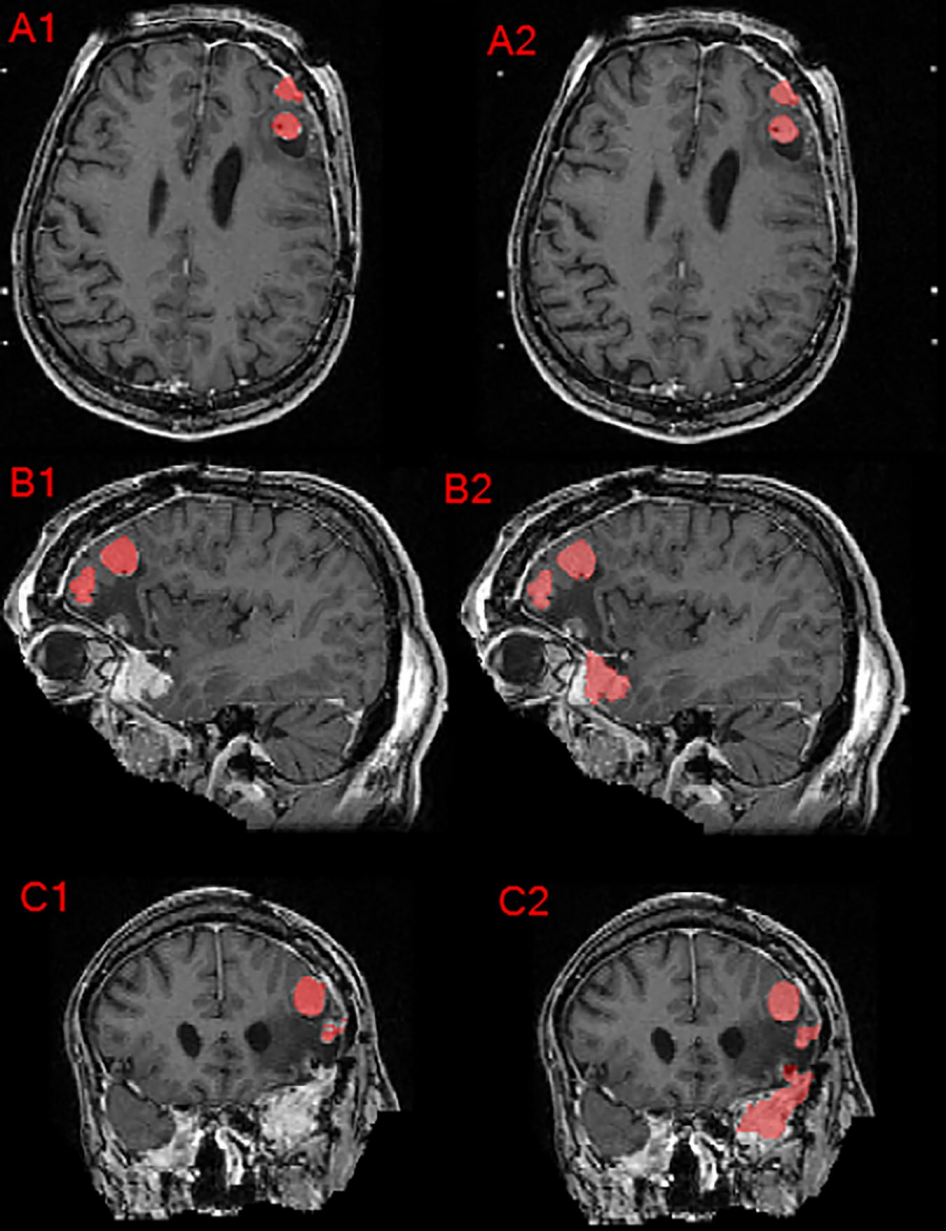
Axial, sagittal, and coronal brain MRI of a patient with multiple meningioma demonstrating the difference between the provided institution’s GTV as seen in panels A1, B1, and C1 compared to the manually revised target label as seen in panels A2, B2, and C2. Note that this case’s corrections involved inclusion of additional meningioma, correction of label edges, and inter-axial slice label smoothening.
